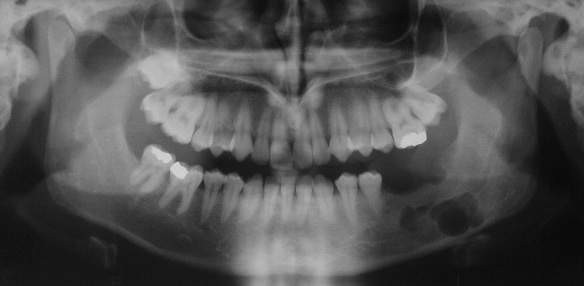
panoramic radiograph showing left mandibular lesion in patient n°1.
